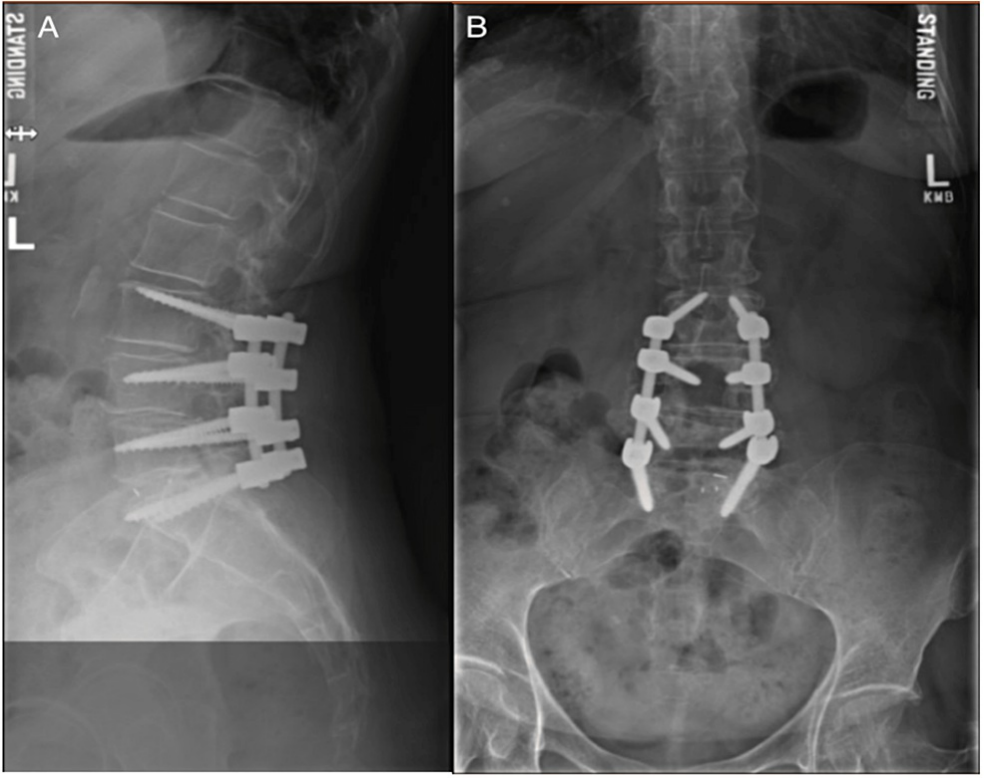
Postoperative X-ray: (A) sagittal standing lumbar X-ray showcasing the implanted spinal hardware; (B) coronal standing lumbar X-ray showcasing implanted spinal hardware.
